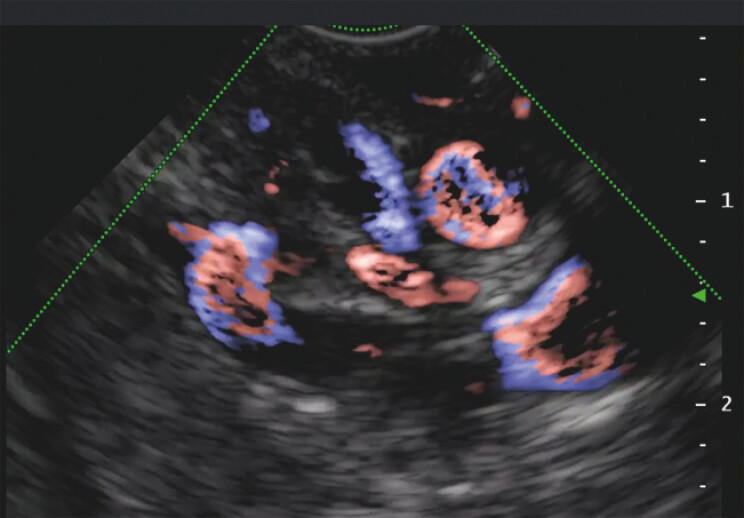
Endoscopic ultrasonography image showing the varicose veins around the anastomosis.
